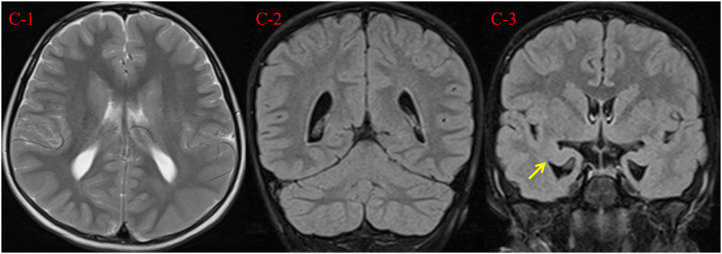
Brain MRI findings at 7 years and 2 months. (C-1, C-2) Axial T2 weighted image and coronal T2 FLAIR demonstrate mild enlargement of the lateral ventricles. (C-3) Coronal T2 FLAIR demonstrates reduced volume of the bilateral hippocampus (arrow), with peculiar temporal horn dilatation.
